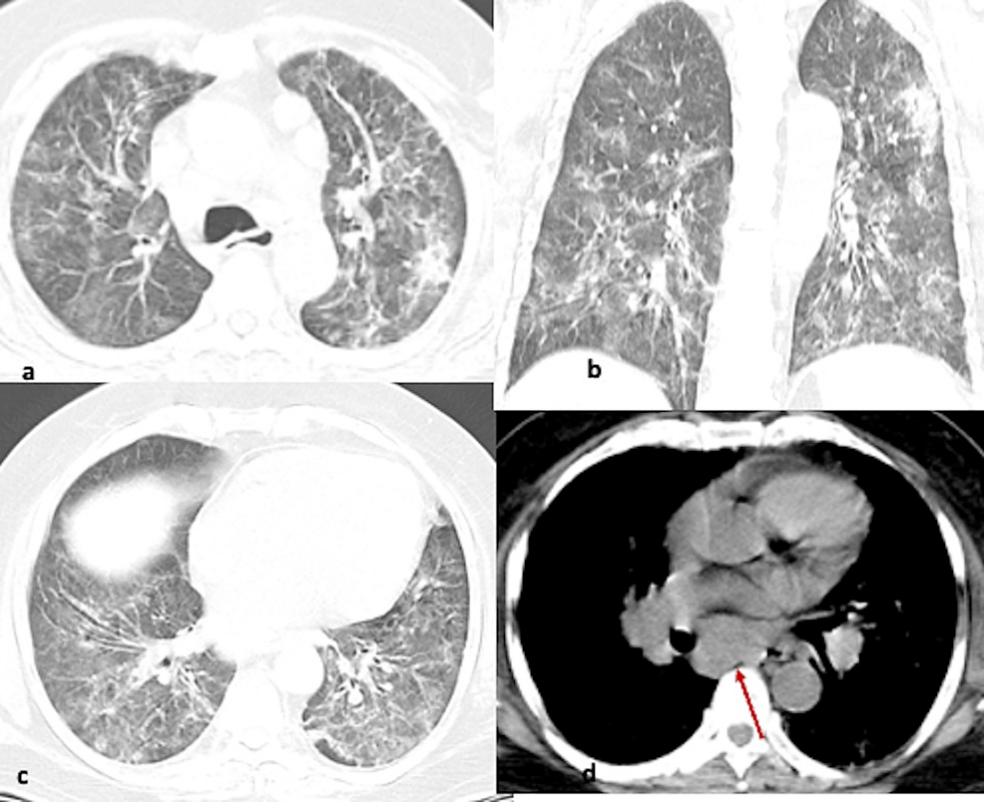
(a-d) Axial and coronal CT chest images in lung and mediastinal window. Extensively diffuse ground glass opacities throughout both the lungs. Small left upper lobe peripheral consolidation. There are significantly enlarged hilar and subcarinal lymph nodes (arrow) which resolved on 6months follow-up.
